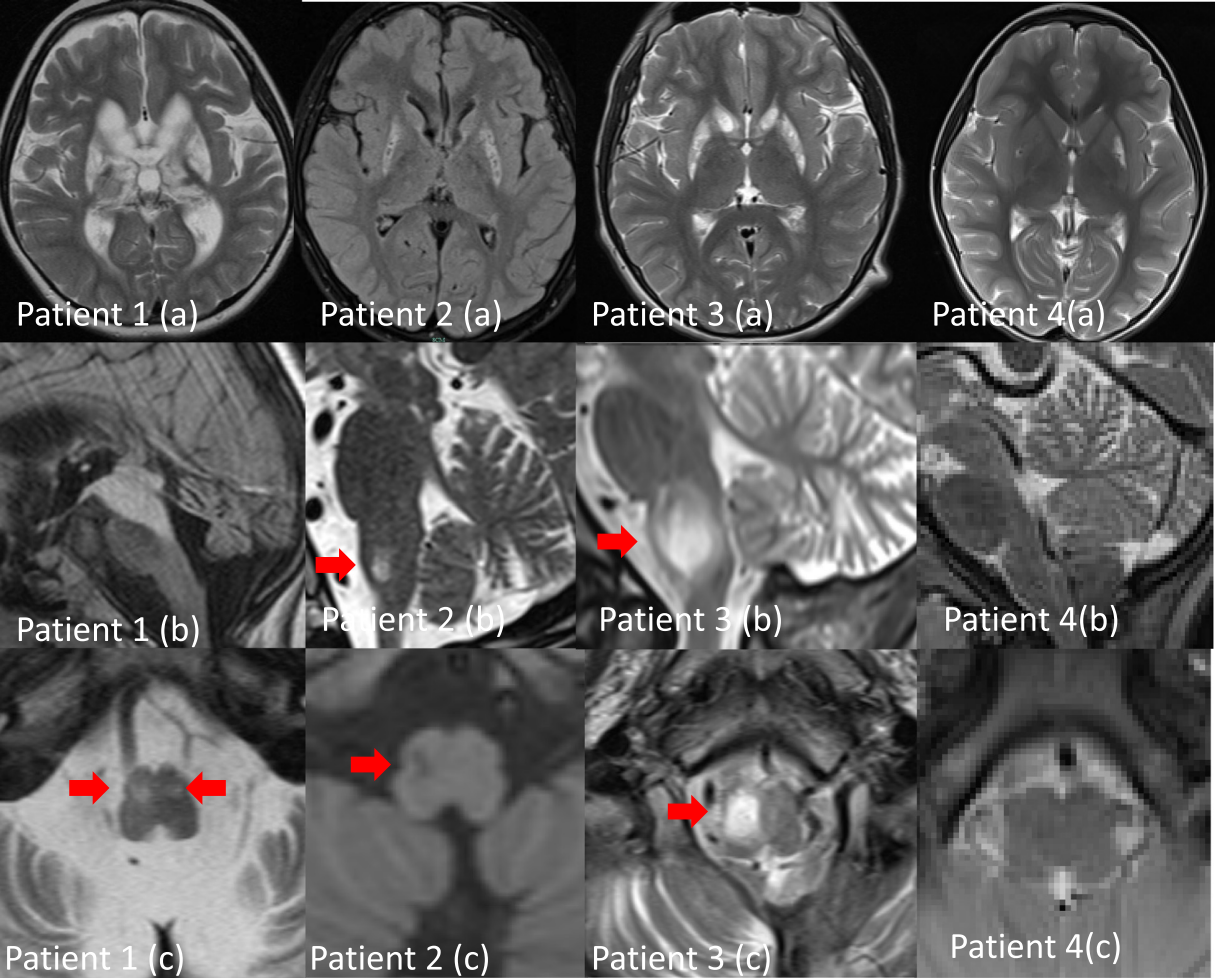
Brain magnetic resonance imaging of the four patients. Hyperintensities in T2 (Flair 2a, 1b)-weighted images indicate pathology of basal ganglia (1a, 2a, 3a, 4a), thalamus (1a), and mesencephalon (1b). Cerebellar atrophy is shown on in 1b, 2b, 3b. The increased interfoliar spaces in 4b may be interpreted as early sign of cerebellar atrophy in the clinical context of patient 4. Hypertrophic degeneration of olivary nuclei are marked with red arrows in 1c, 2b and c (T1 signal hypointensity), 3b and c. It is not seen in 4b, c.
